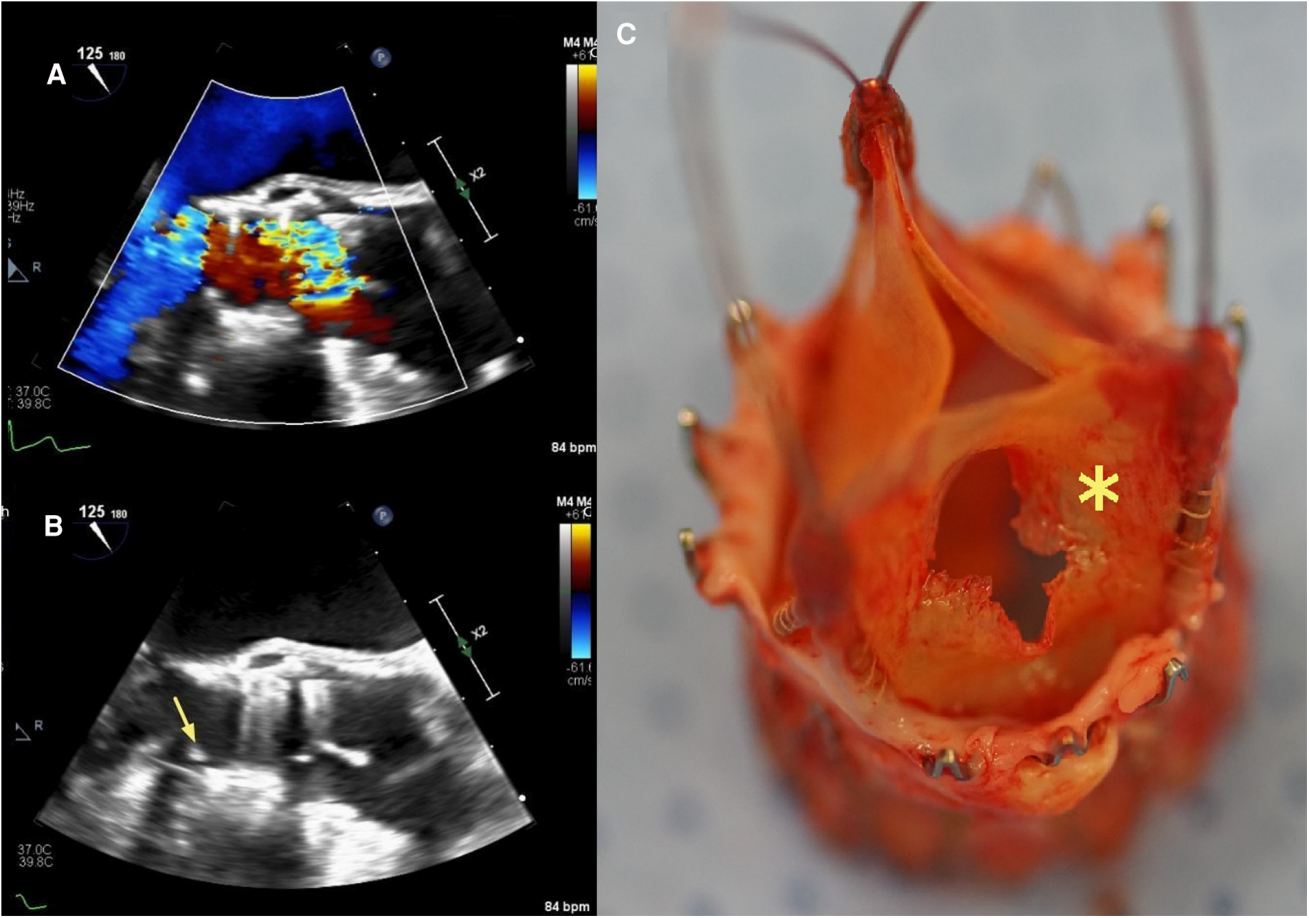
Case 2: transesophageal echocardiography three-chamber view with color-flow Doppler (A) and aortic valve-centered short-axis view with color-flow Doppler (B) showing severe intraprosthetic regurgitation and partial cusp prolapse (yellow arrow) on the right coronary side, respectively. (C) Explanted ACURATE Neo prosthesis with a central tear in the leaflet in the right-coronary position (yellow asterisk).
